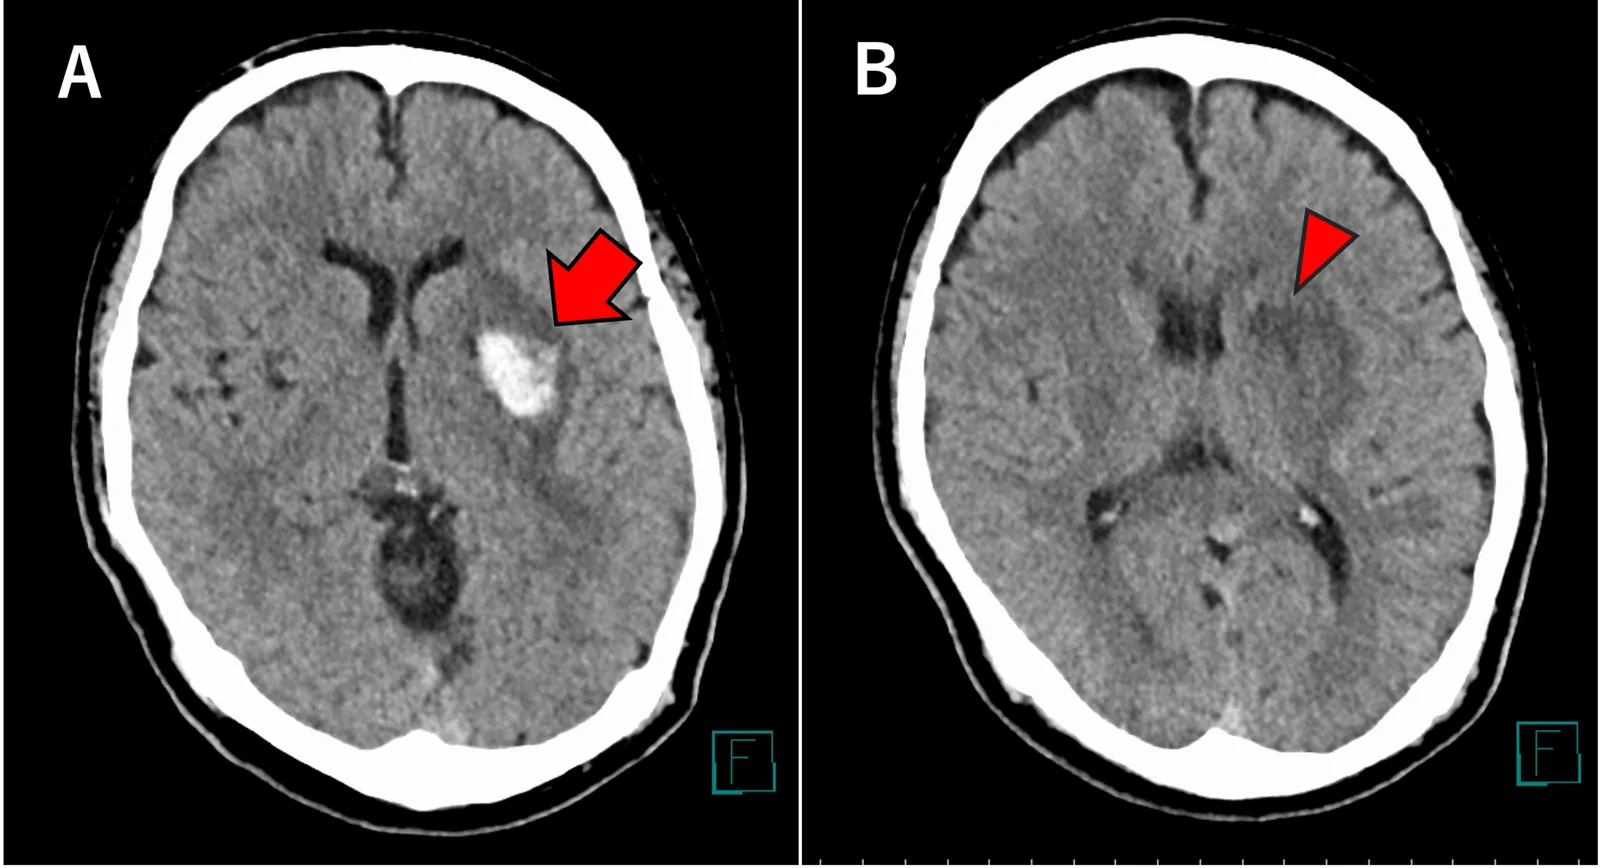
Initial non-contrast computed tomography of the head. (A) Axial computed tomography showed a left putaminal hematoma with surrounding edema (arrow). (B) A slightly more cranial slice showed a subtle hypoattenuating area adjacent to the hematoma, extending toward the left corona radiata within the lenticulostriate artery territory (arrowhead).
